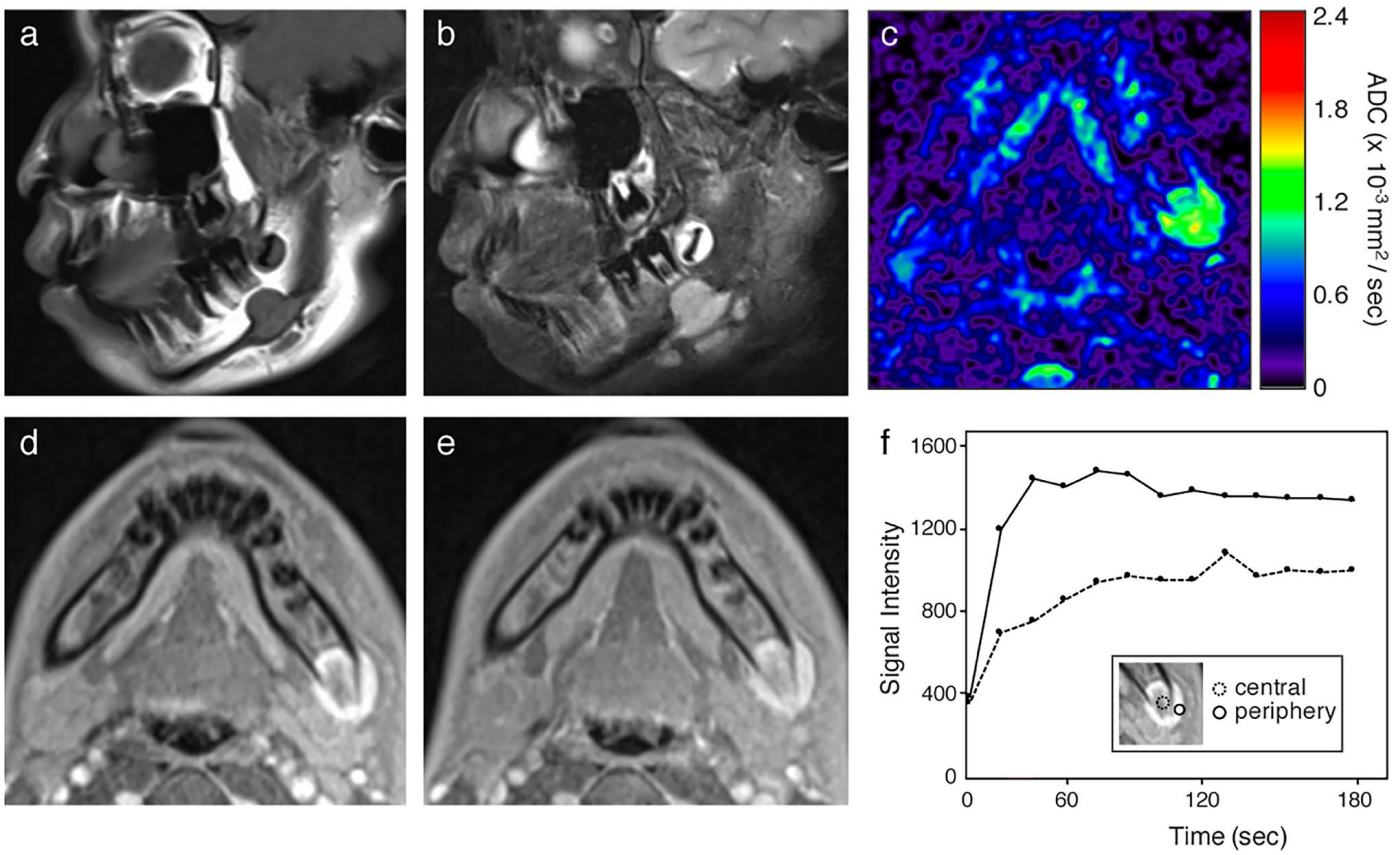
Magnetic resonance imaging of the lesion in the left mandible. a Sagittal T1-weighted image showing a well-defined mass with homogeneous low signal intensity. b Sagittal fat-suppressed T2-weighted image showing a mass with heterogeneous high signal intensity. c Lesion exhibiting moderate apparent diffusion coefficient (ADC; 1.2 × 10^−3^ mm^2^/s), as calculated by diffusion-weighted imaging using two b-values of 500 s/mm^2^ and 1000 s/mm^2^. d Dynamic contrast-enhanced image 30 s after the injection of the contrast agent. e Dynamic contrast-enhanced image 180 s after the injection of the contrast agent. f Time-intensity curves showing a gradual increment pattern in the central region of the mass and a rapid uptake followed by a gradual decrement pattern in the peripheral region of the mass
